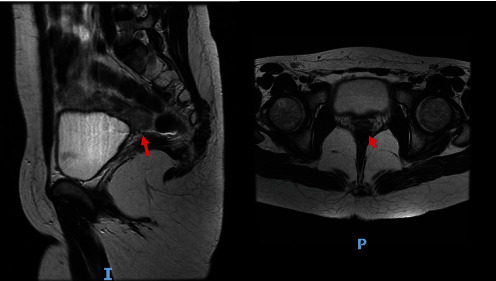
Magnetic resonance imaging (MRI) shows the presence of the lower two-thirds of the vagina were observed with the absence of Müllerian structures.
